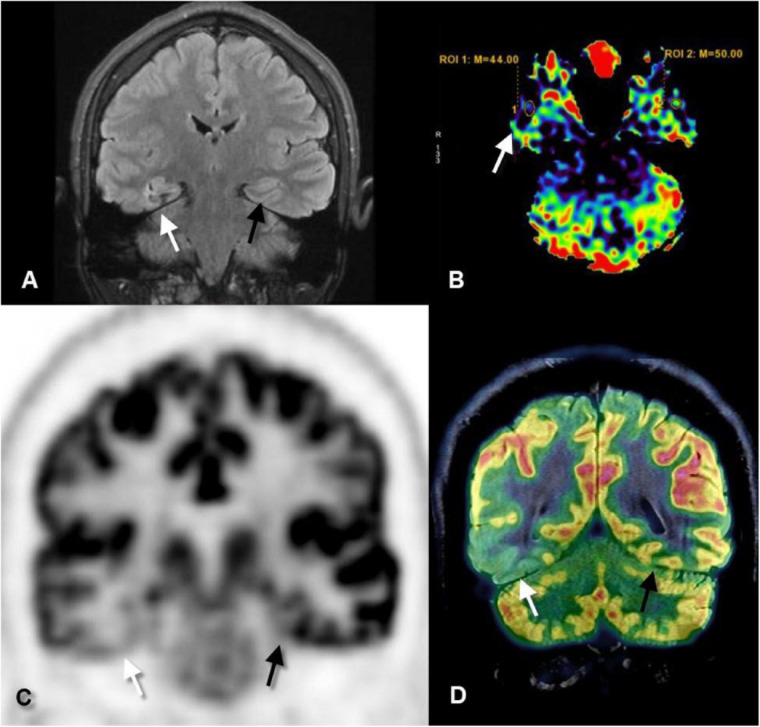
Coronal fluid-attenuated inversion recovery (FLAIR) (A), axial perfusion-weighted imaging-arterial spin-labeled (PWI-ASL) (B), coronal positron emission tomography (PET) (C), and coronal PET–MRI fusion (D) images of a 23-year-old male with longstanding epilepsy and underwent the scans in the interictal period. FLAIR image revealed mild increased signal and mild gliotic changes of the right hippocampus (white arrows) as compared to the left one (black arrows). PWI-ASL revealed a decrease in the cerebral blood flow (CBF) in the right hippocampus [region of interest (ROI) 1 = 44] as compared to the left hippocampus (ROI 2 = 50) denoting hypoperfusion. PET and PET–MRI images revealed pronounced hypometabolism of the right temporal lobe particularly the hippocampal formation as compared to the contralateral side. Quantitative data showed standardized uptake value (SUV) of right hippocampus measured 2.56 while on the left hippocampus measured 4.88 (Case courtesy of Prof. Dr. Yasser Abdel Azeem, Ain Shams University).
